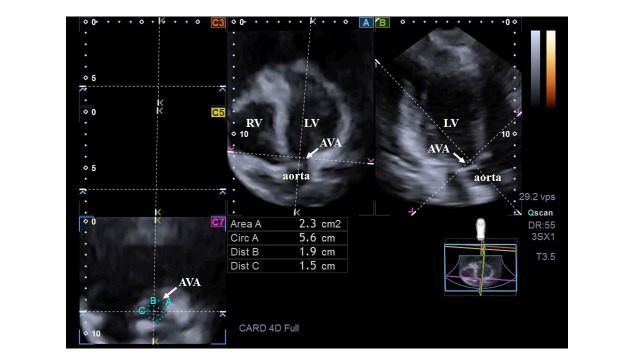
Evaluation of aortic valve annular geometry via three-dimensional speckle-tracking echocardiography. Assessment of aortic valve annular (AVA) dimensions in apical four-chamber (A) and two-chamber (B) long-axis views by three-dimensional speckle-tracking echocardiography. ‘En-face’ view of the AVA is also presented in C7 cross-sectional view. Abbreviations: LV, left ventricle; Dist B and C, maximum and minimum AVA diameters; Circ, AVA perimeter; area, AVA area; AVA, aortic valve annulus.
